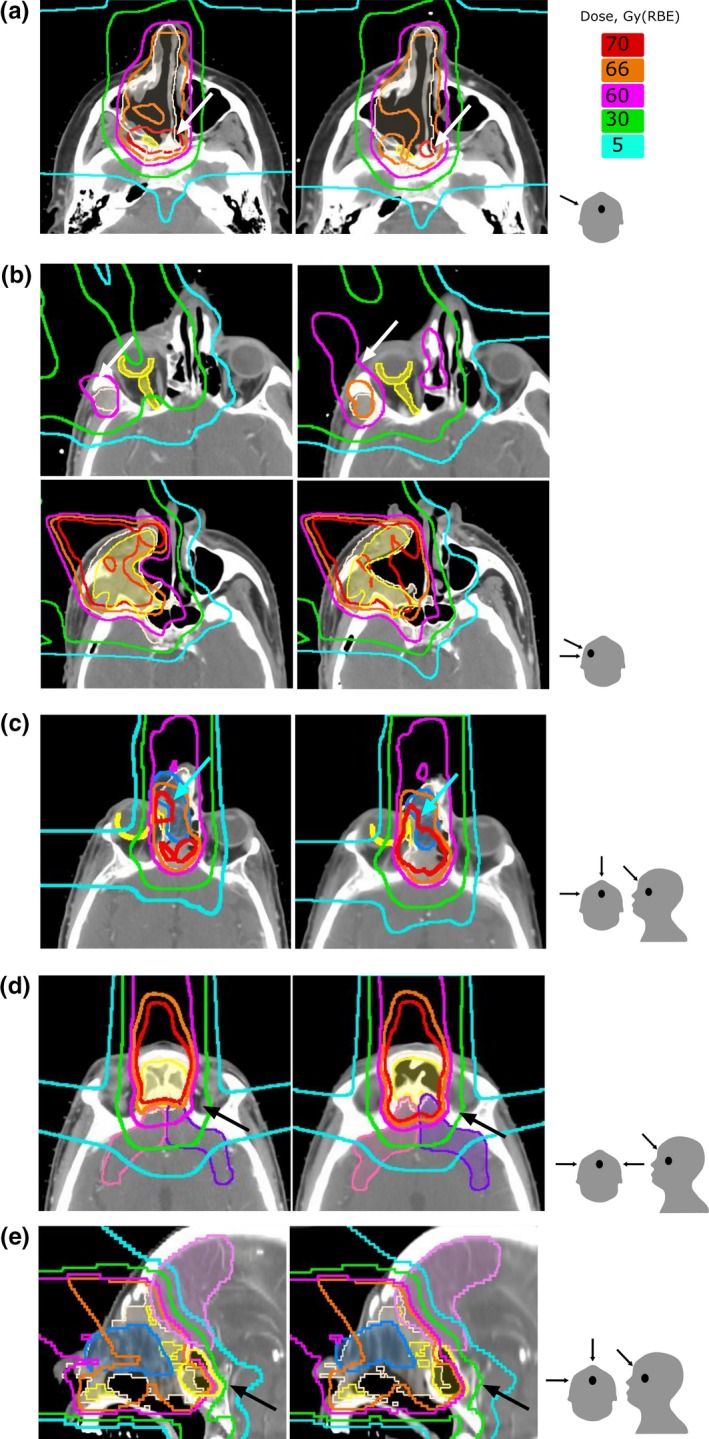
Comparison of dose distributions calculated on planning CT (left) and repeat CT (right). gross tumor volume (GTV) and CTV are shown in shaded yellow and white color, respectively. Panel (a) shows aeration increase in the right maxillary sinus and a shift of the dose away from GTV (patient #12). Panel (b) (upper row) shows an increased dose to the right retina and optic nerve. Panel (b) (lower row) shows decrease in GTV and CTV coverage due to increase in soft tissue in the posterior wall of the right maxillary sinus (patient #5). Panel (c) shows increase in the dose to the right retina due to shrinkage of the surgical flap (patient #1). Panel (d) shows increase in the dose to the frontal lobes due to clearing of the bilateral frontal sinus (patient #14). Panel (e) shows increase in the dose to a frontal lobe due to fluid clearing in the sphenoid sinus and shrinkage of the surgical flap (patient #1). The last column demonstrates the direction of the boost fields.
